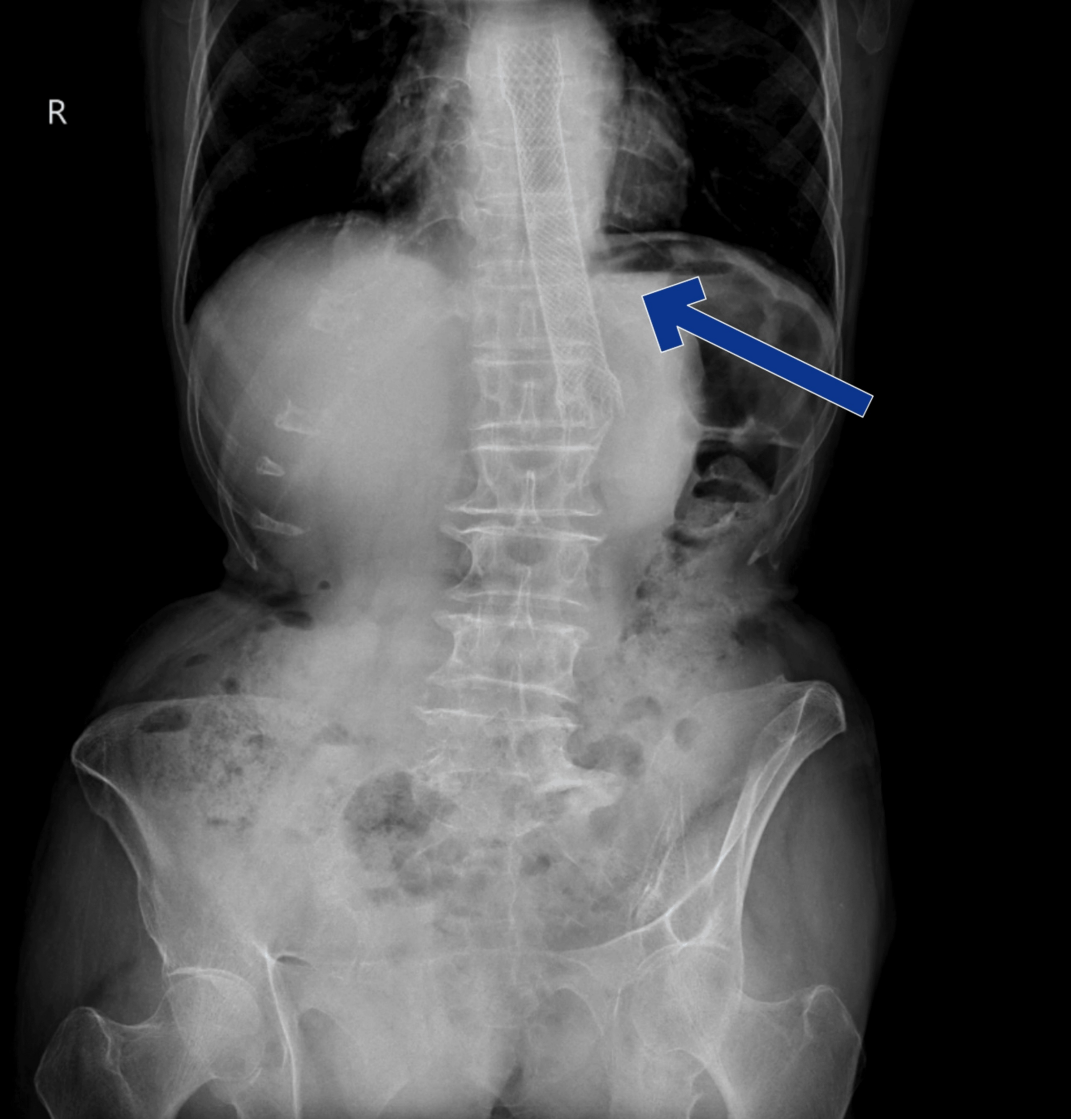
X-ray posterior-anterior view of the thorax showing the distally migrated metallic stent (blue arrow)
